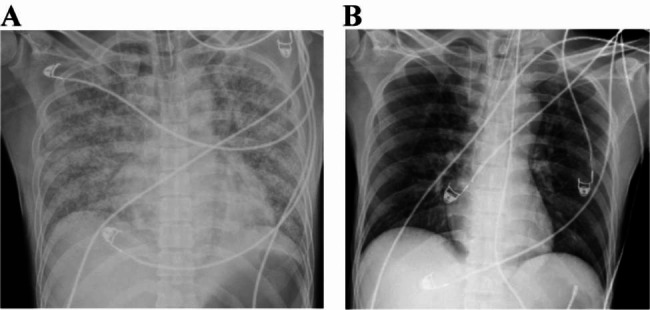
The changes in pulmonary X-ray before and after ECMO support
